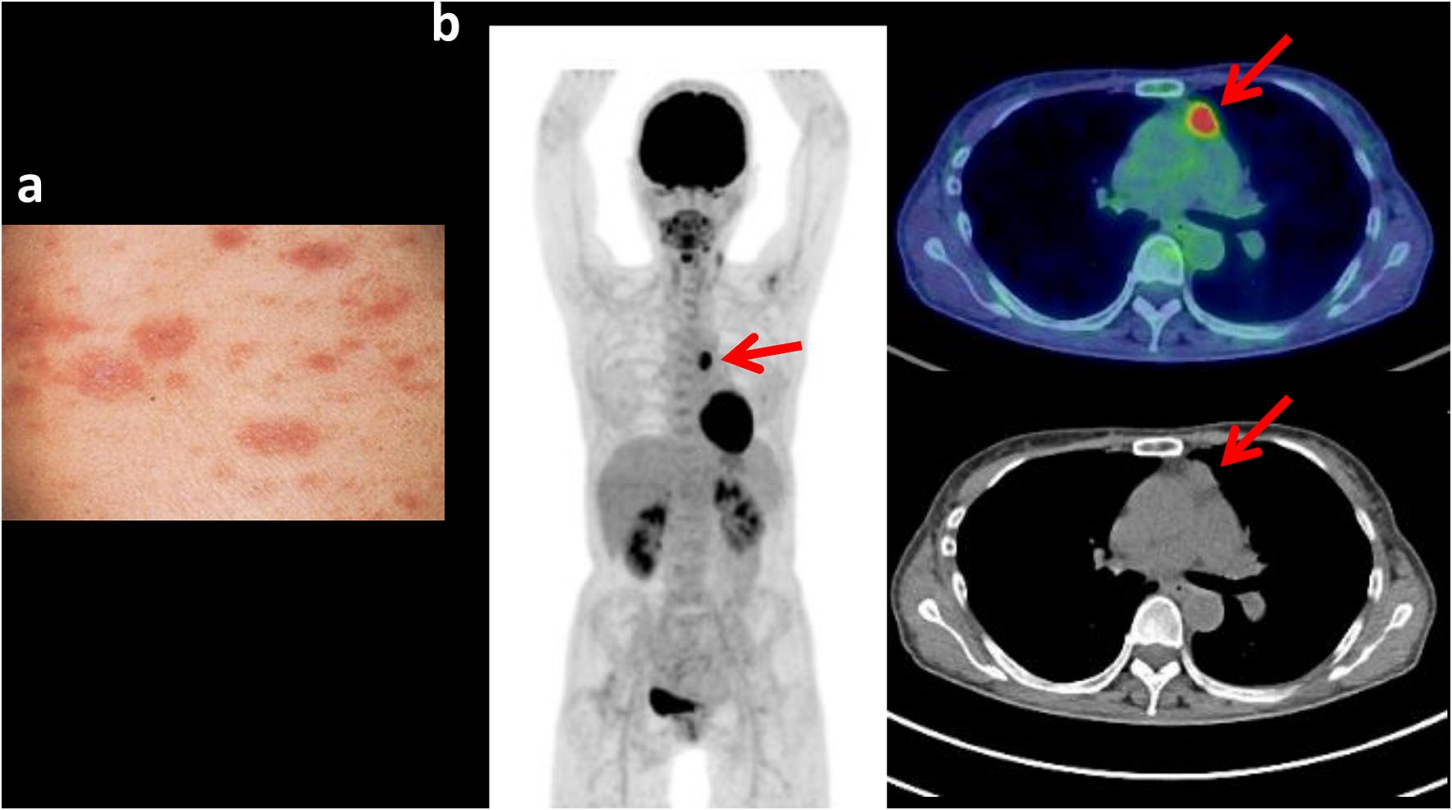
Close-up image of cutaneous lesion of lichen planus in the abdominal wall in a 75-year-old female (a). The cutaneous lesions began as erythematous patches on her hands and then spread to her feet, arms and legs, and trunk. Whole-body FDG PET maximum projection and cross-sectional images showed FDG-avid (SUVmax 7.4) mass in the right upper mediastinum (b; arrows), which was later diagnosed as thymic cancer by surgical resection. The cutaneous lichen planus lesions did not show any increased FDG uptake probably due to the thin and tiny lesions
